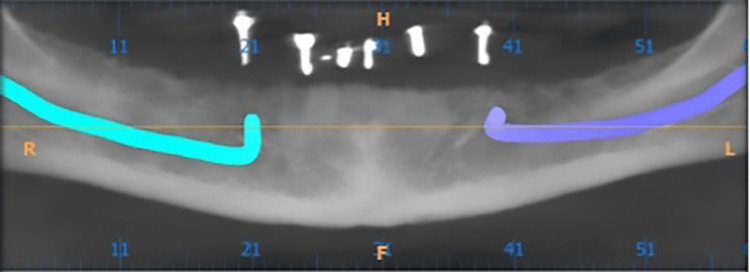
Cone beam computed tomography (CBCT) with radiographic marker. Image Credit: Sourabh Khandelwal
